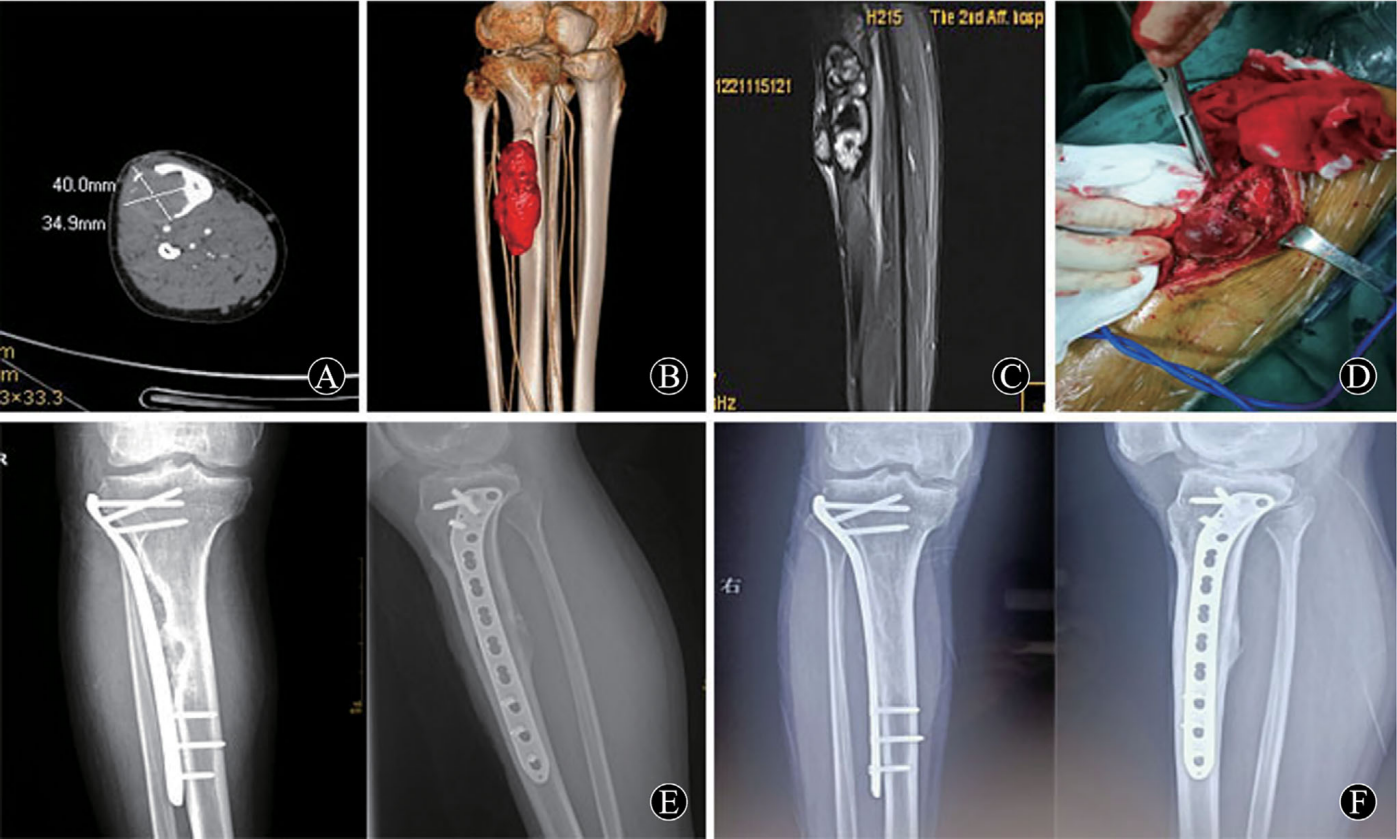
A 32‐year‐old man (patient 8) who had a 5‐year history of right lower leg progressive swelling and pain. His diagnosis was a hemophilic pseudotumor in tibia. (A) Preoperative CT image showed bone destruction. (B) Three‐dimensional CT has an important role in determining the shape and size of pseudotumor. (C) Preoperative MRI T2 image in the coronal plane, which showed heterogeneous signal intensity. (D) Intraoperative photograph showing exposure of pseudotumor. (E) Postoperative X‐ray taken at 1 month after surgery, allograft and internal fixation were performed after excision of pseudotumor. (F) Postoperative X‐ray taken at 25 months after surgery, the bone defect healed.
